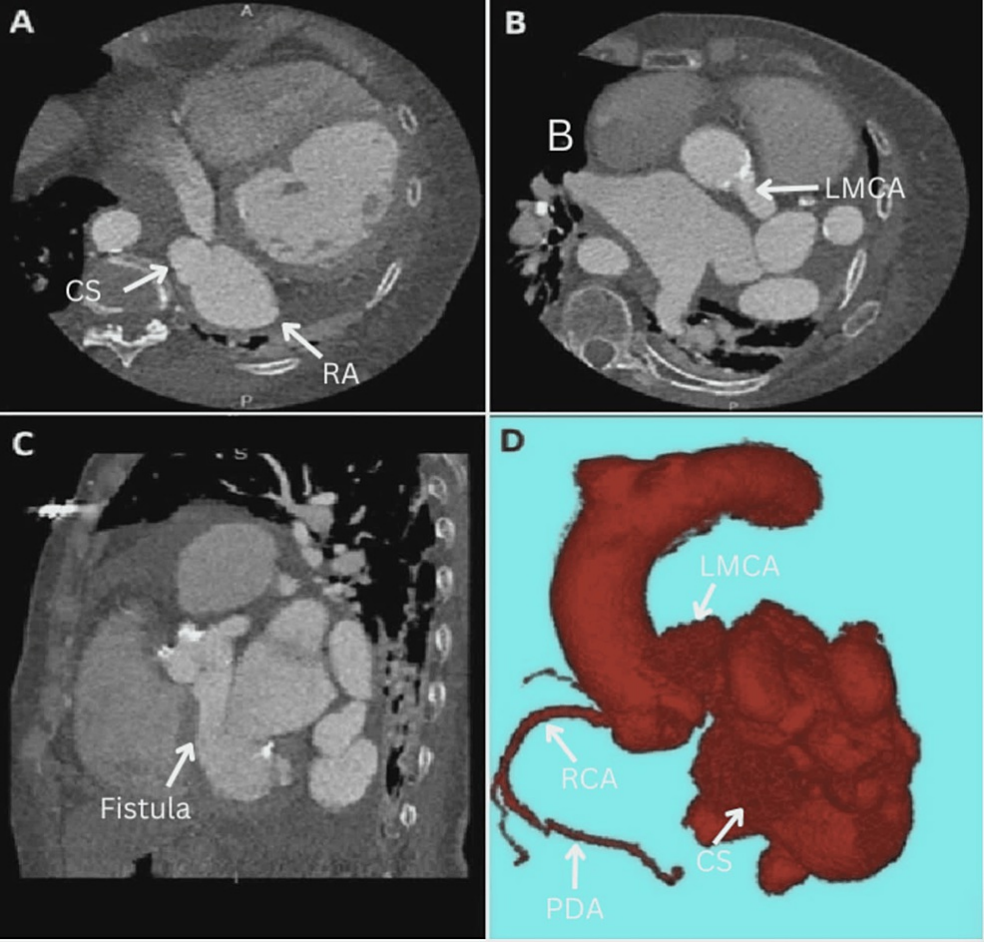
(A-C) Cardiac CTA. IV contrast is noted filling the right atrium during this arterial-timed contrast bolus study. The anatomy of the left coronary artery and circumflex is unable to be interpreted. (D) 3D CTA rendering. The coronaries arise from a normal position. There is right dominance. The left main coronary artery arises from the left coronary cusp and appears markedly dilated and tortuous. There is a large cavernous and partly calcified coronary arteriovenous fistula from the left main artery that appears to drain to the right atrium via a dilated coronary sinus. Multiple loops and segments of this large dilated fistula are noted along the lateral side of the left atrium and left ventricle as it courses toward the coronary sinus and right atrium. LMCA: Left main coronary artery; CTA: Computerized tomography angiography.
